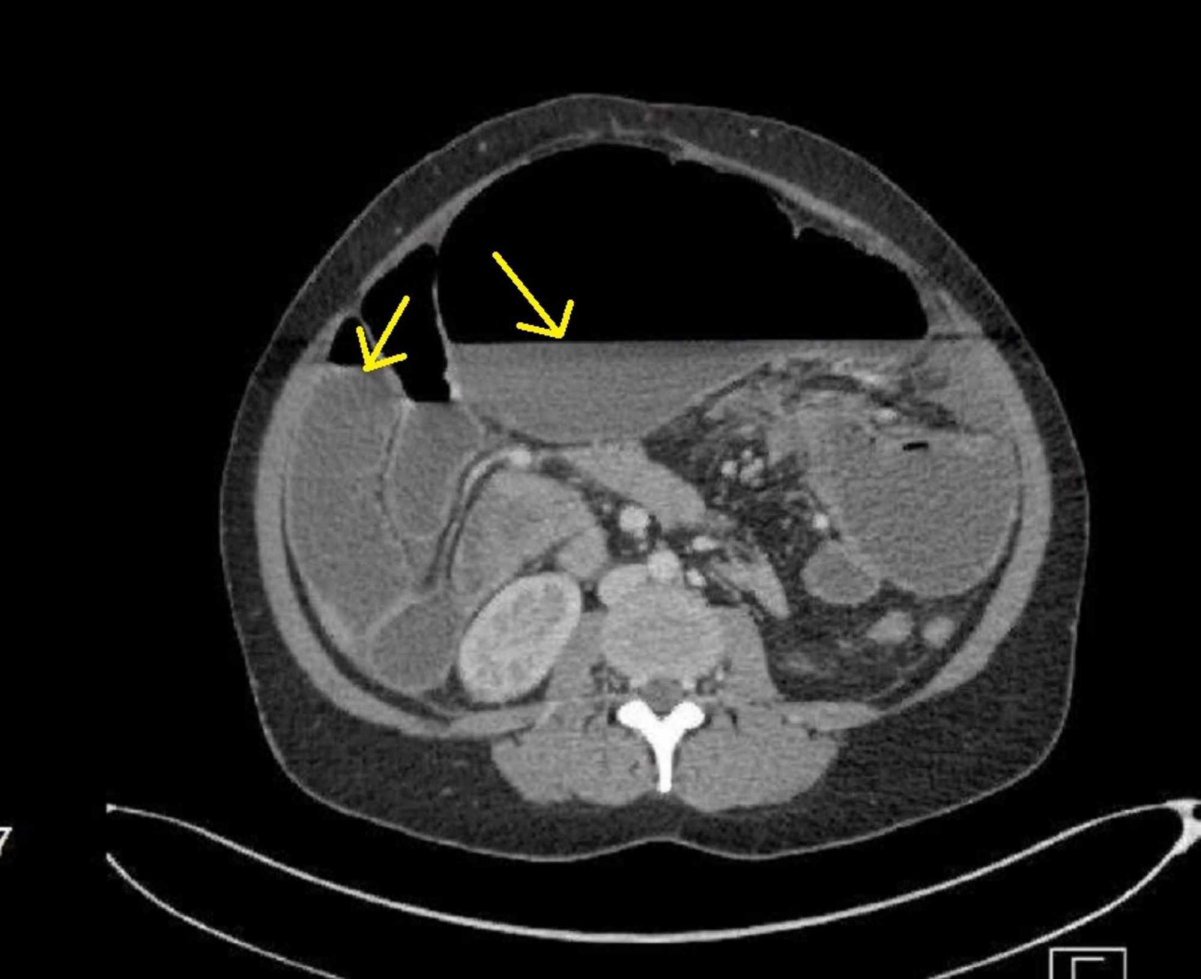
Abdominal computed tomography with contrast revealing the dilated bowel loop and air-fluid
